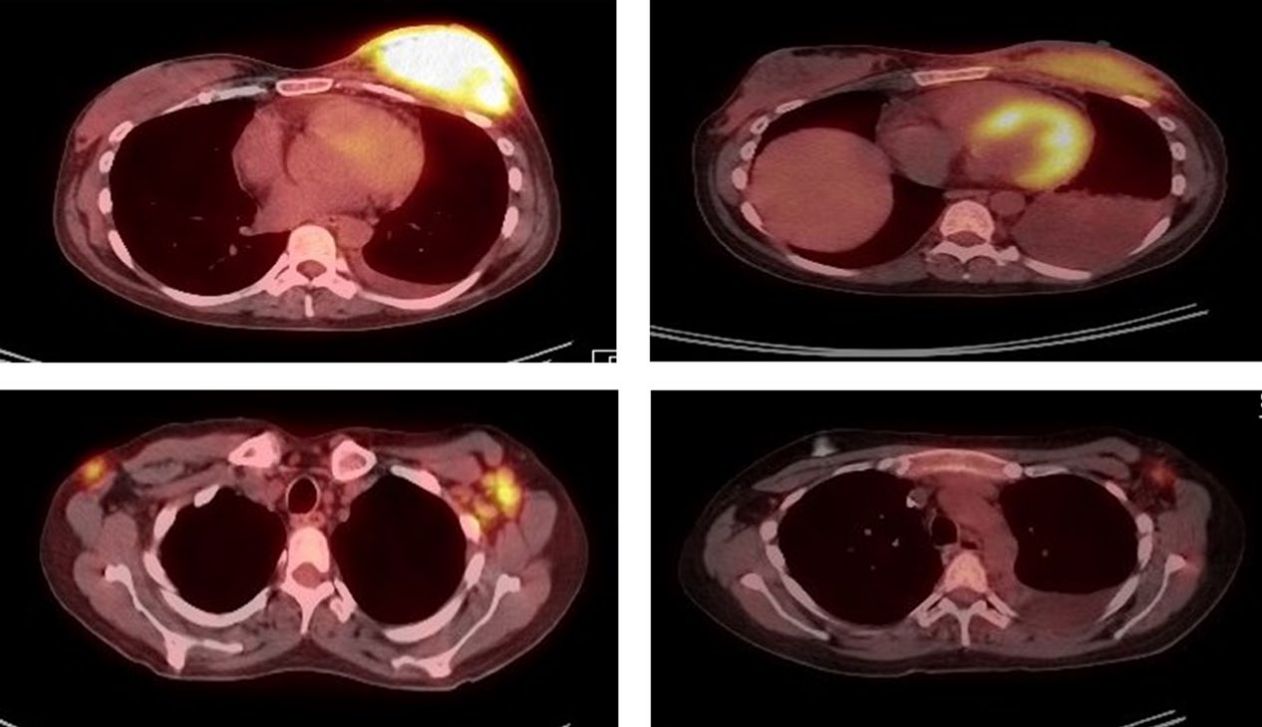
PET-CT at diagnosis and following 6 cycles of chemotherapy. Left column: PET-CT at diagnosis prior to chemotherapy. Right column: PET-CT following 6 cycles of chemotherapy.
